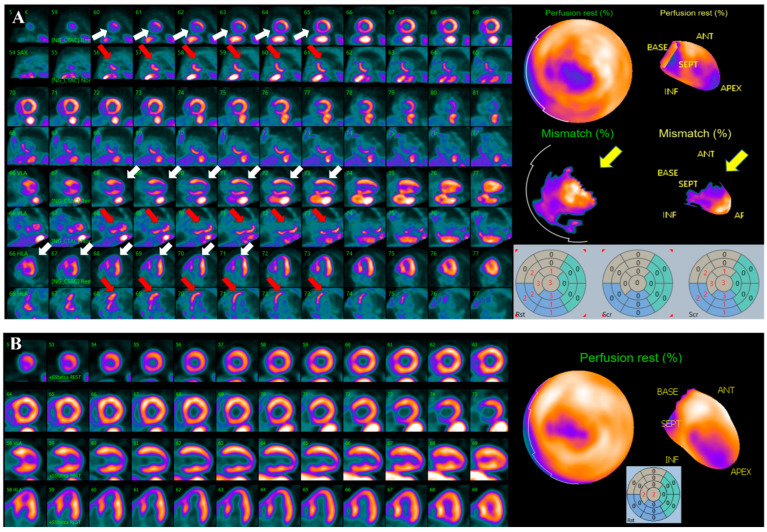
Courtesy of Prof. Wanda Acampa, Dept. of Advanced Biomedical Sciences, University of Naples “Federico II”. (A) Combined perfusion/metabolism evaluation by rest 82-Rubidium and 18F-FDG PET-CT in a 74-years old female patient, experiencing an ACS 1-year post-CABG on LAD and OM, with apex and infero-septal wall akinesia and severe LVEF reduction assessed by echocardiography, along with sub-occlusive RCA stenosis and graft degeneration identified by ICA. Upper lines show LV perfusion; bottom ones show metabolic assessment. White arrows indicate a large, fixed perfusion defect involving apex and infero-septal region, red arrows indicate significant glucose uptake in these regions, yellow arrows indicate the perfusion/metabolism mismatch, suggestive for the presence of hibernating/viable myocardium, involving more than 20% of LV, and worth further revascularization. (B) Rest 82Rb PET-CT performed 1 year after PCI on RCA showing substantial improvement in myocardial perfusion. Echocardiography report also documented significant motion function and LVEF improvement.
